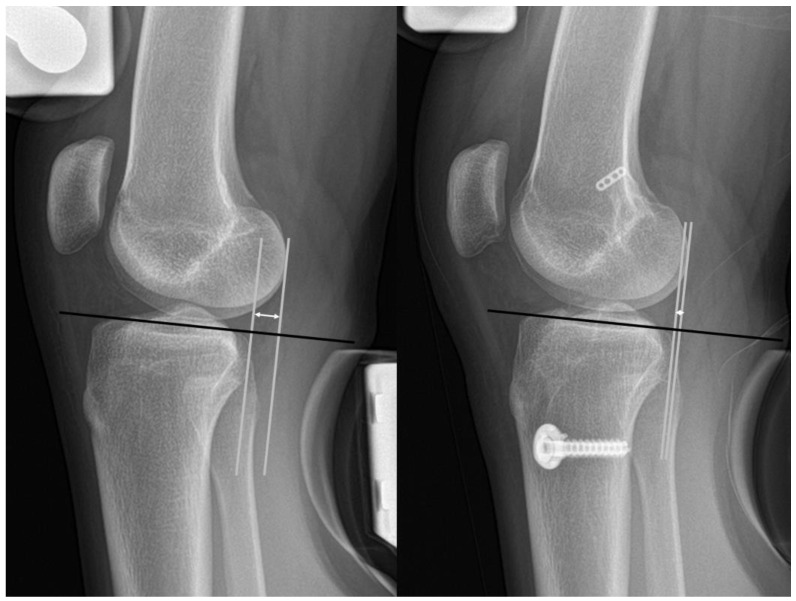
Measurement of pre- and postoperative anterior tibial translation on Telos stress radiographs. A reference line (black line) was drawn parallel to the tibial plateau joint line. Perpendicular lines (white lines) from the reference line were drawn tangentially to the most posterior contour of the femoral condyle and tibial plateau. The anterior tibial translation was defined as the distance between two lines (arrow line).
